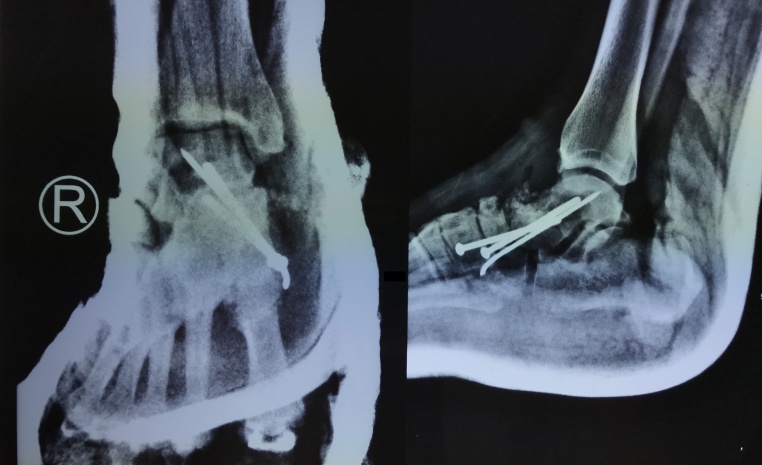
Plain radiograph after forefoot arthrodesis procedure fixed by screws and K-wire.
